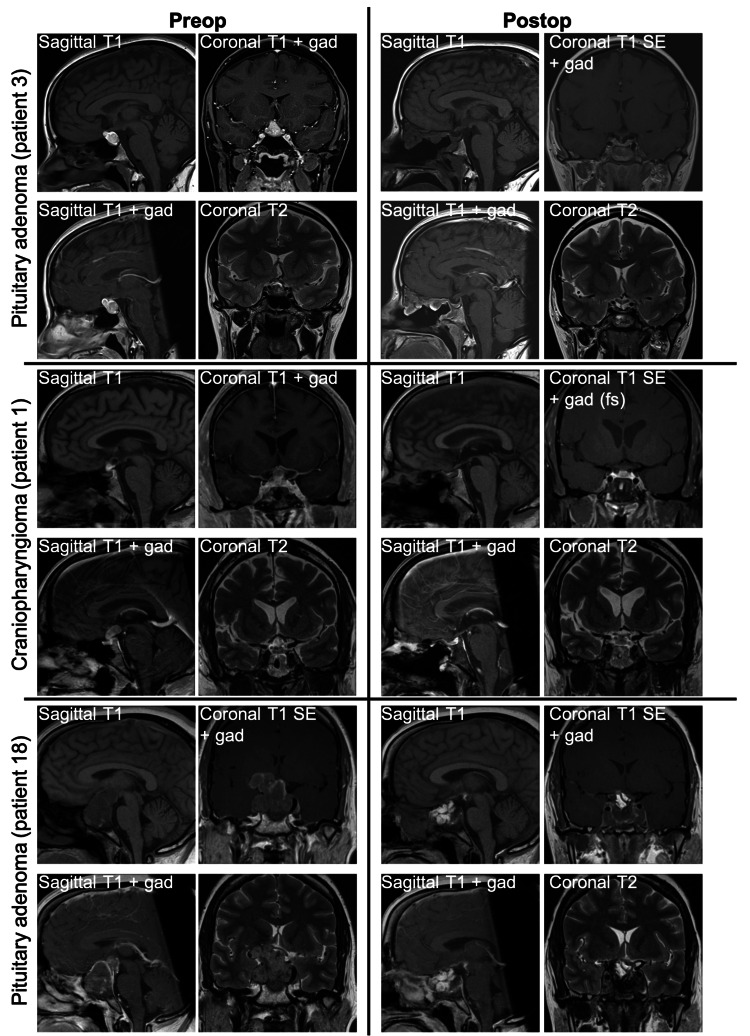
Examples of surgical outcomes achieved using EndoSNAP Preoperative and postoperative MRI images are displayed. The T1 hyperintensities observed in the surgical cavity of patient 18 on postoperative images indicate the presence of a fat graft. EndoSNAP, Endoscopic Surgical Navigation Advanced Platform; fs, fat suppression; gad, gadolinium; SE, spin echo
